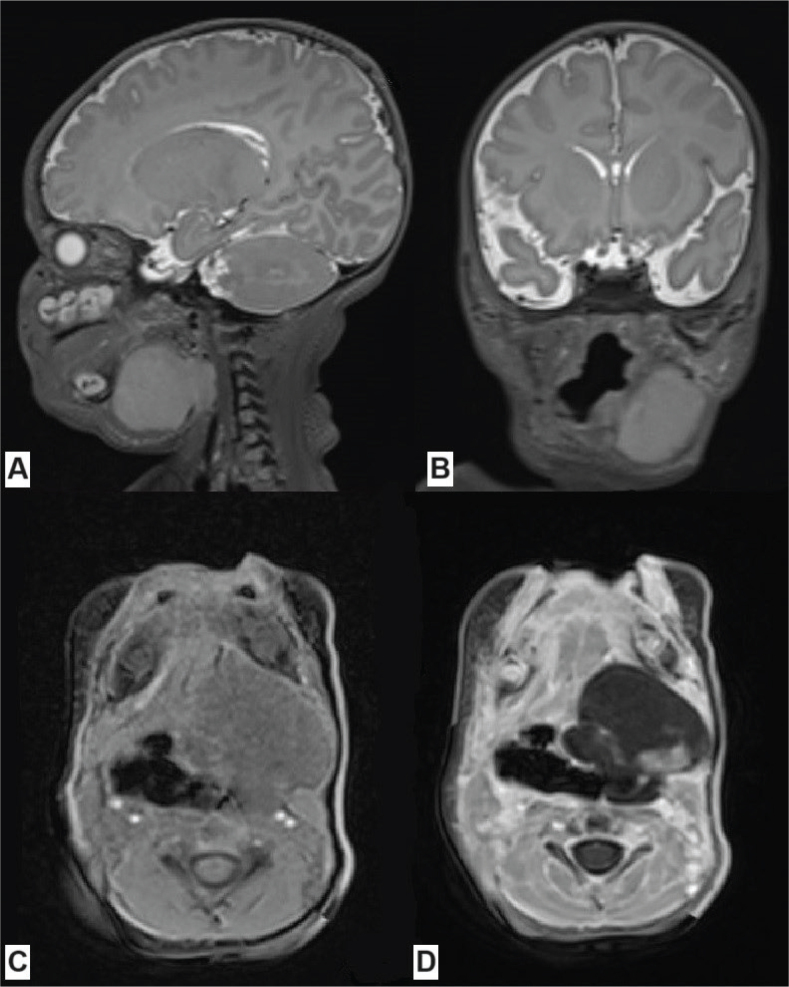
Craniofacial MRI of patient #2 at the time of diagnosis showing the tumor in the left submandibular region, modeling and displacing the left salivary gland, the styloglossus muscle, the posterior belly of the digastric muscle, the root of the tongue, the muscles of the mouth floor, the oral and laryngeal parts of the pharynx, and downwardly reaching the level of the middle part of the larynx: (A) T2 SPACE sequence (sagittal), (B) T2 SPACE sequence (coronal), (C) T1 VIBE sequence (axial), (D) T1 VIBE contrast enhanced sequence (axial). MRI: Magnetic Resonance Imaging.
